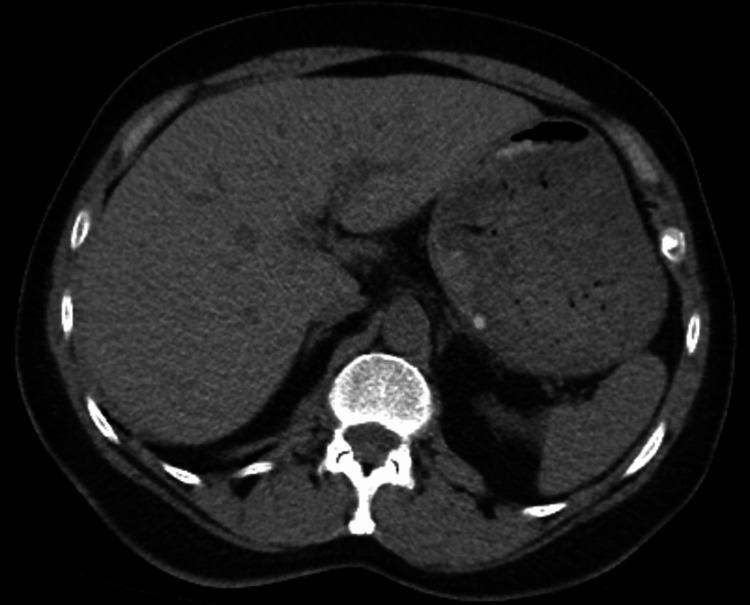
Hospital Day 1: CT abdomen and pelvis without contrast
